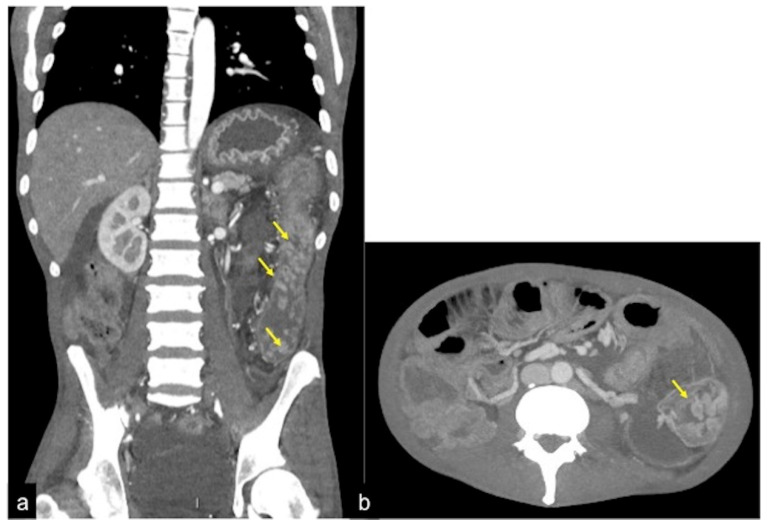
CTA arterial phase (a) and relative MIP reconstruction (b) of polyposis of the descending colon (a,b, arrows).
